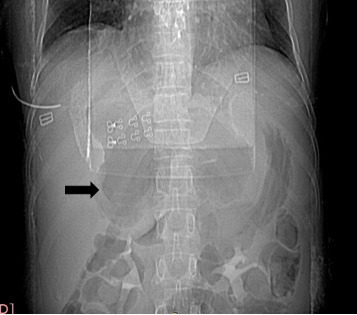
X-ray of the abdomen without preparation: Large extra luminal gas collection
